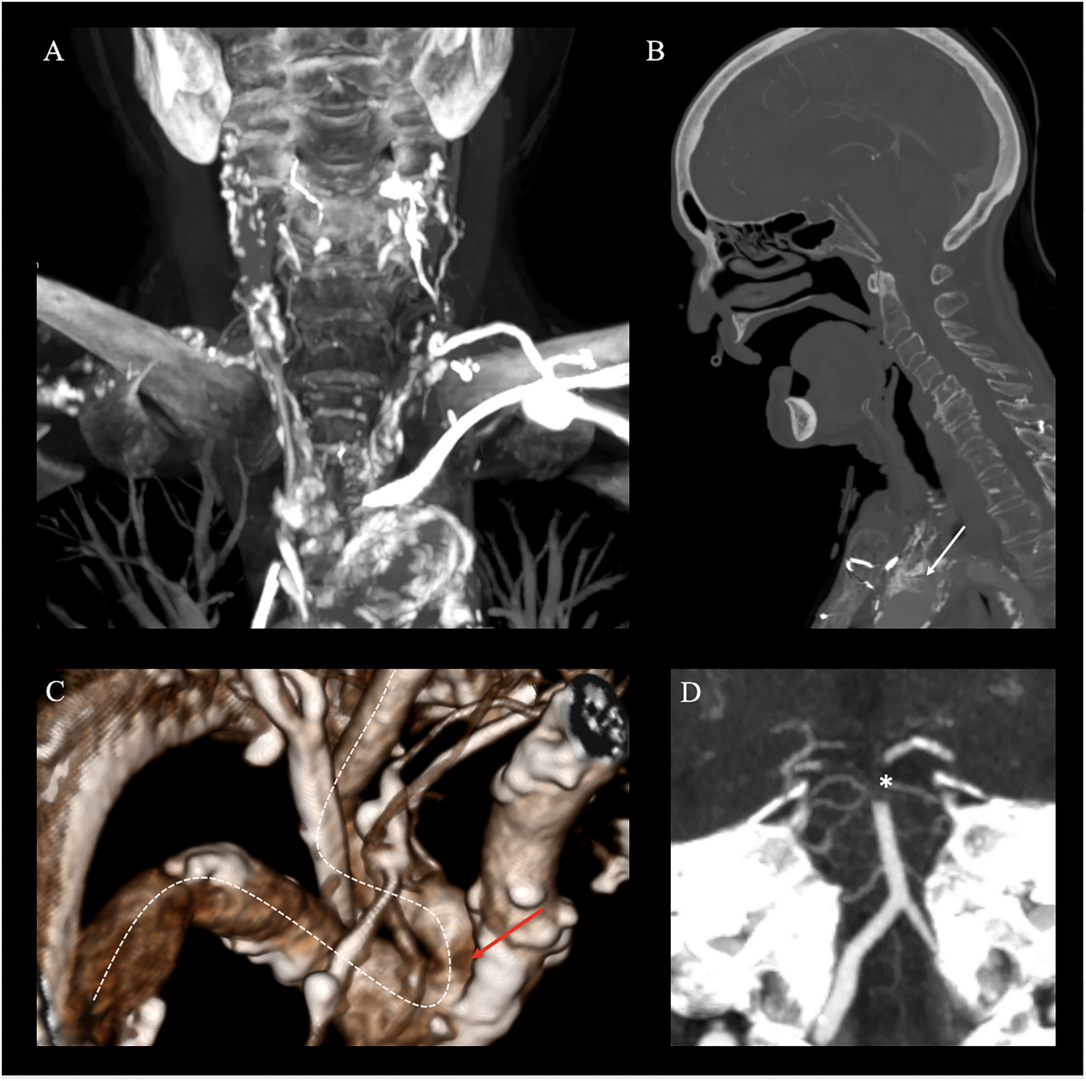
Non-invasive emergency imaging. Emergency imaging of a 70-year-old female patient with acute basilary artery occlusion (BAO), on. (A–D) Computed Tomography Angiography (CTA) in MIP reconstruction shows severe atherosclerosis of the aorta and cervical arteries (coronal plane), (A) a prolapsing stent of the common carotid artery into the aortic arch (white arrow, sagittal plane), (B) kinking and stenosis of the right subclavian artery (white dotted line) and an osteal right VA stenosis on the right (red arrow) with a steep-angled take-off from the subclavian artery (white dotted line, 3D reconstruction), (C) and distal occlusion of the basilary artery extending to both P1 segments of the posterior cerebral artery [*; white star, paracoronal plane, (D)].
